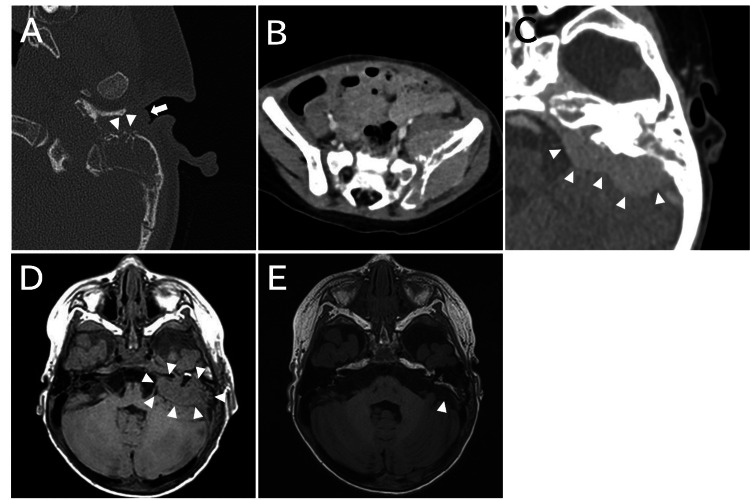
Pre- and post-treatment imaging. (A) Pretreatment axial CT scan of the temporal bone. The soft tissue fills the mastoid cavity, the posterior wall of the external auditory canal is destroyed (arrowheads), and the soft tissue extends into the external auditory canal (arrow); (B) Pretreatment axial enhanced CT scan of the left atrium. Bone destruction of the left ilium and a tumor lesion around the left ilium are observed (arrowheads); (C) Pretreatment axial enhanced CT of the head. A tumor lesion is observed in the posterior cranial fossa; (D) Pretreatment axial T1-weighted MRI of the head. A tumor lesion extending from the left petrous bone to the cerebellopontine angle is observed (arrowheads). Brain metastases are not observed. (E) Post-treatment axial T1-weighted MRI of the head shows tumor shrinkage (arrowhead).
